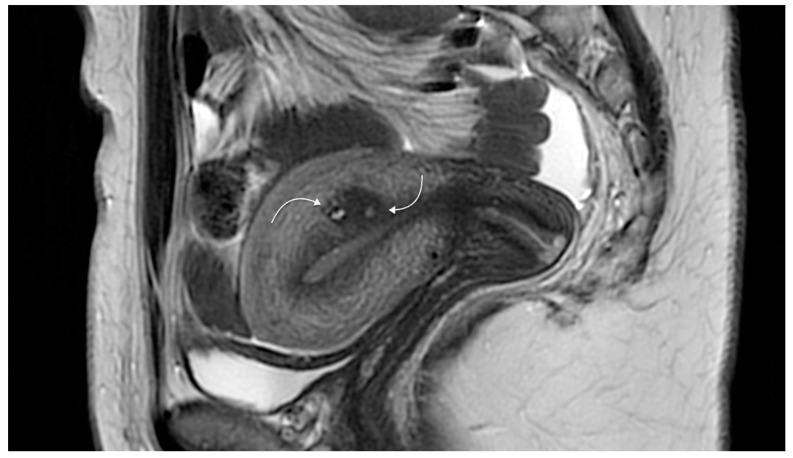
Magnetic resonance imaging showing sagittal two-dimensional T2-weighted magnetic resonance images showing a hypointense lesion containing tiny spots (curved arrows) and located in the posterior wall, adjacent to the endometrial cavity related to focal adenomyosis. Reproduced with permission from Habiba et al. (2020).
